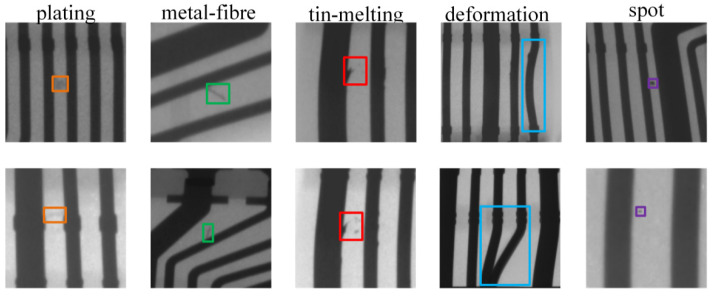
Examples of common types of defects in the insert molding under X-ray.
